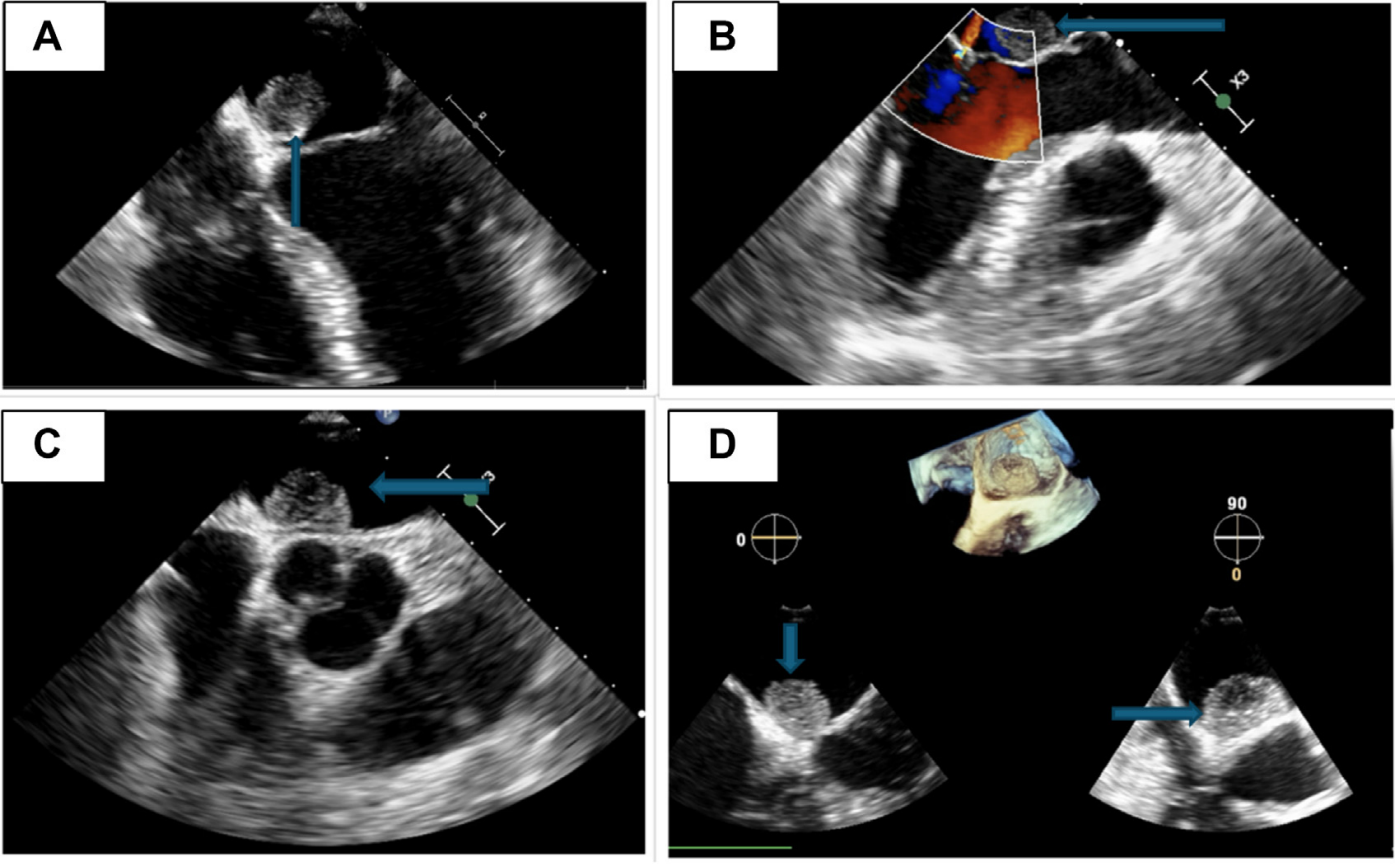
Transesophageal Echocardiogram Transesophageal echocardiogram reveals a 2.3 × 1.7 cm mass in the left atrium (A), indicated by an arrow, consistent with a myxoma. The mass is attached to the interatrial septum (B) and is positioned anteriorly near the aortic valve (C), as shown by arrows. It appears broad-based without a distinct stalk and is located approximately 1 cm above the mitral annulus (D), with no evidence of obstruction to mitral inflow.
